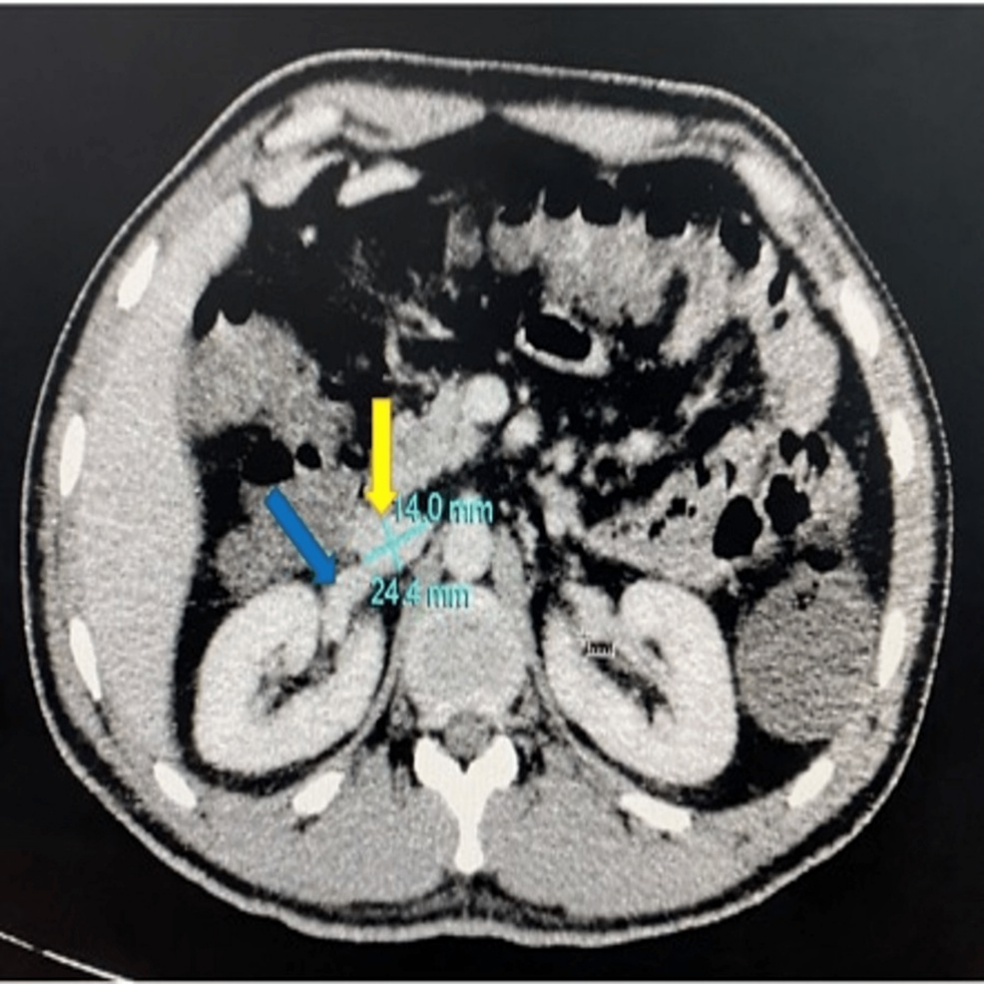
Axial CT scan image showing an inferior vena cava (yellow arrow) measurement taken at the level of the renal vein (blue arrow)
